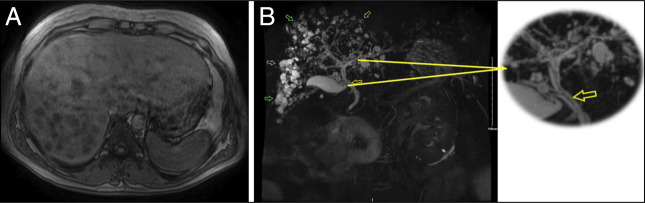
Magnetic resonance imaging with magnetic resonance cholangiopancreatography of the abdomen axial section (A) and coronal section (B) shows innumerable hyperintense and hypointense lesions with peripheral enhancement (green open arrow) and linear T2-hypointense structure in the RHD, CHD, CBD (yellow open arrow). CBD, common bile duct; CHD, common hepatic duct; RHD, right hepatic duct.
